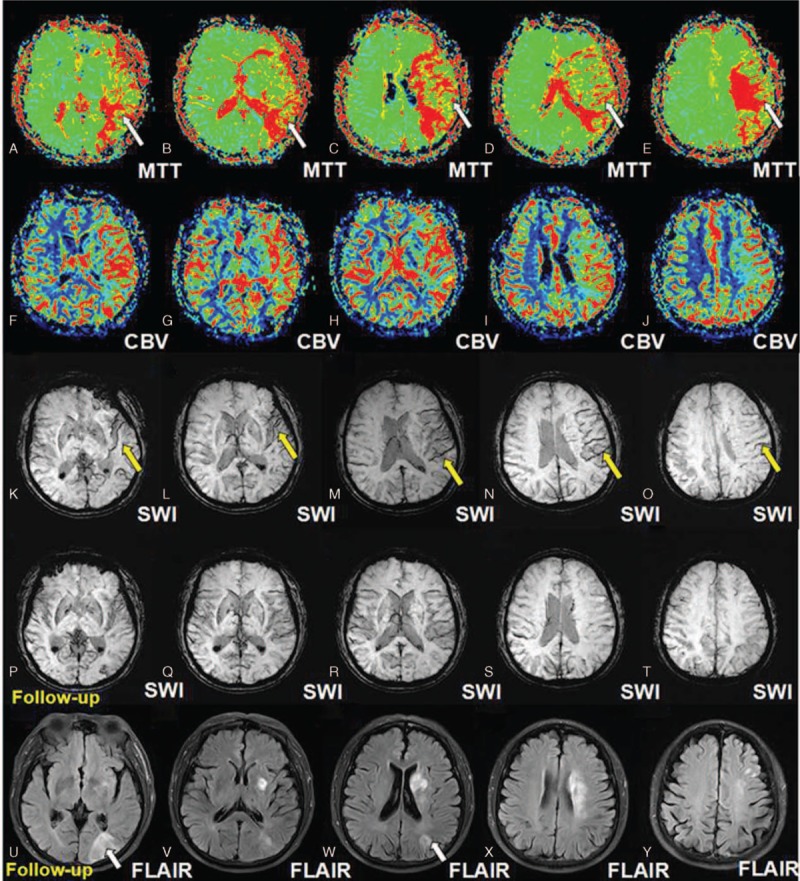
Perfusion weighted imaging and susceptibility weighted imaging and follow-up imaging. A–E (arrows) indicated the more prolonged MTT on PWI of the affected MCA and F–J showed elevated CBV of the affected MCA. In addition, the K–O (arrows) revealed ACVS or PV on SWI in the affected MCA territory, which disappeared in the follow-up SWI, as revealed by P–T. The mismatch between DWI-PWI and DWI-SWI, indicating the ischemic penumbra, was comparable. The expansion of the infarction resulting from the penumbra in the affected MCA territory, as shown on FLAIR image during follow-up indicated by the mismatch between DWI-SWI which was comparable with that of DWI-PWI. ACVS = asymmetrical cortical vessel sign, CBV = cerebral blood volume, DWI = diffusion weighted imaging, FLAIR = fluid attenuated inversion recovery, MTT = mean transit time, PV = prominent vein, PWI = perfusion weighted imaging, SWI = susceptibility-weighted imaging.
